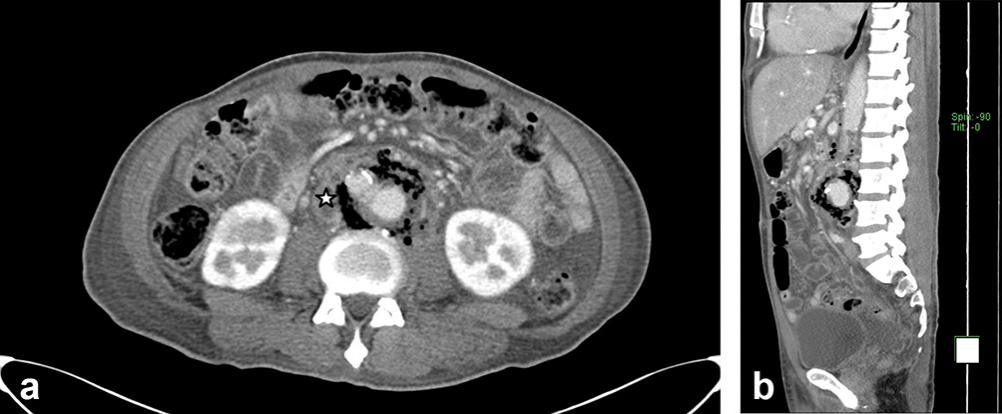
Contrast-enhanced CT images in axial (a) and sagittal (b) sections: a—periaortic collection (star) noted, with associated periaortic air pockets; b—an emphysematous region is noted, in close proximity to the vertebrae, with no evidence of bony destruction.
